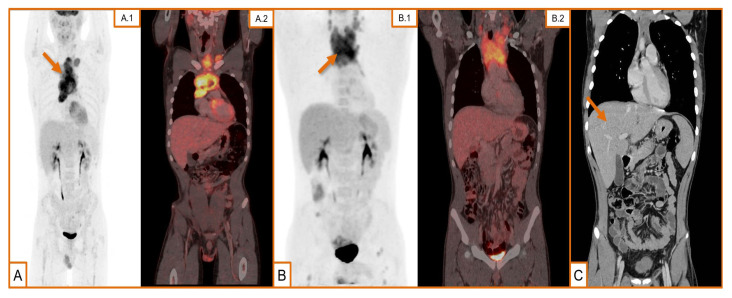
Radiological findings in our case evolution. (A) (A.1) Maximum intensity projection (MIP) image of positron emission tomography (PET) using 18F-Fluorodeoxyglucose (18F-FDG) as radiotracer. Hypercaptant mediastinal mass (arrow) with heterogeneous distribution suggestive of thymoma/lymphoproliferative syndrome at the time of diagnosis; (A.2) fusion images, sagittal axe. (B) (B.1) MIP image of PET using 18F-FDG as tracer. Supradiaphragmatic and cervical adenopathy clusters with a bulky mediastinal mass (arrow) and pathological FDG bone deposits in both femurs during the first relapse; (B.2) fusion images, sagittal axe. (C) Remarkable reduction in the size of the mediastinal mass, although newly observed hepatomegaly (arrow) after salvage therapy.
